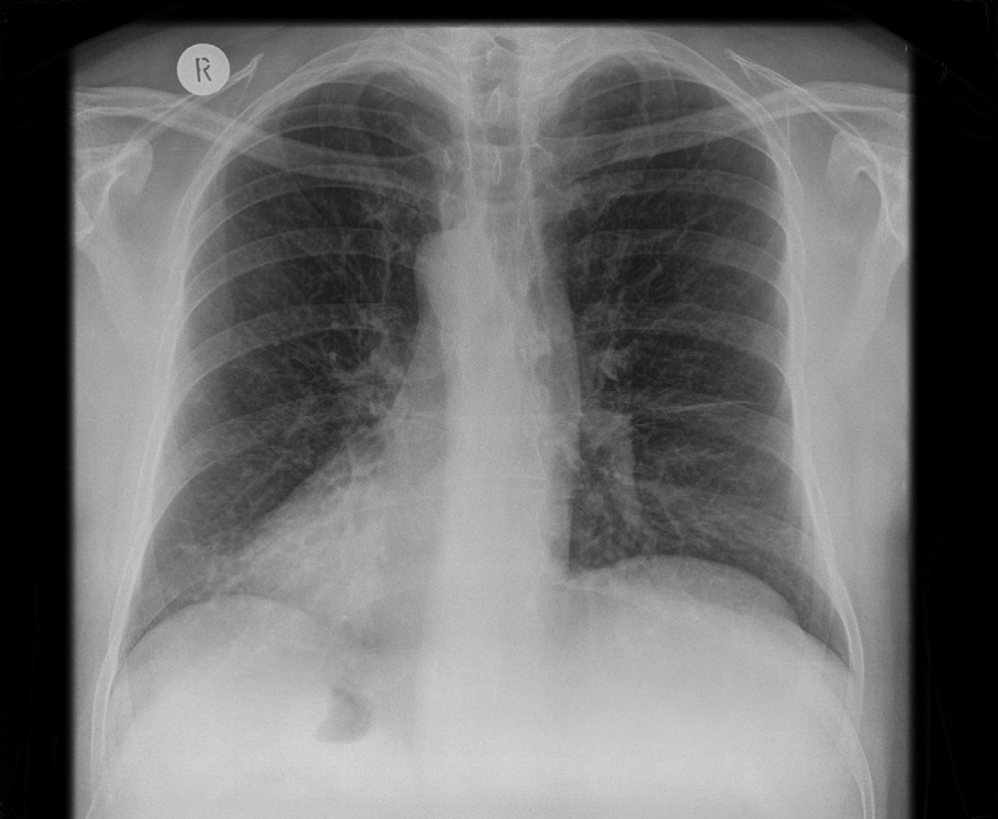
A chest radiograph showing dextrocardia with situs invertus totalis in an individual with PCD.
